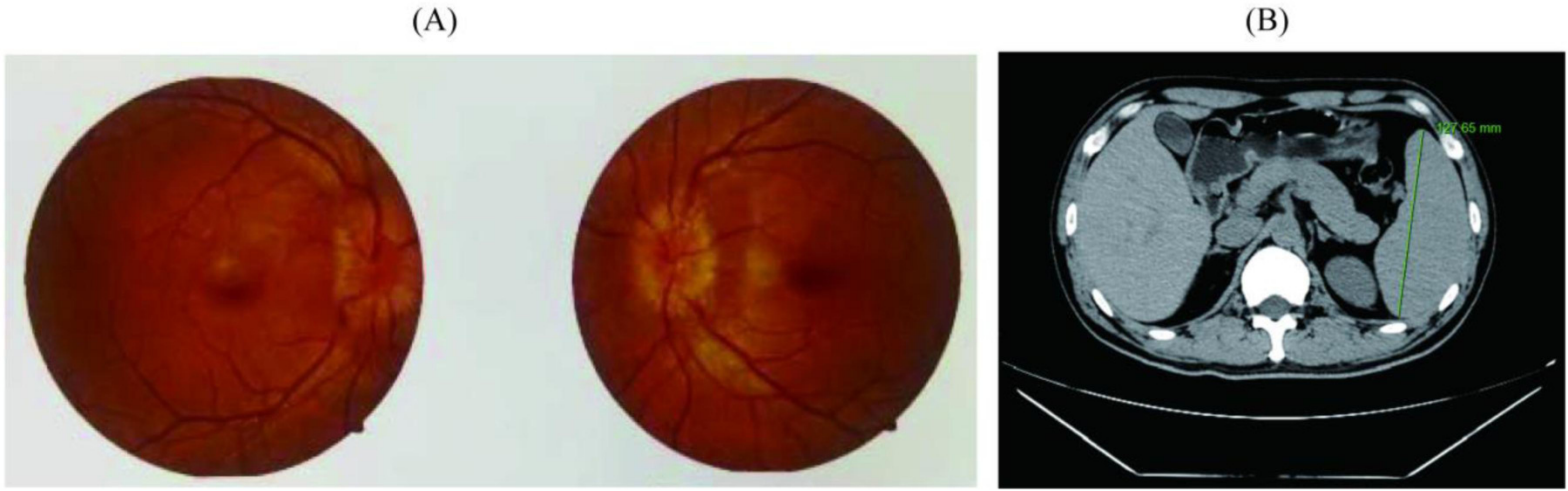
Examination results of patient-related complications. (A) Results of fundus optic nerve examination; (B) Results of spleen computed tomography scan.
